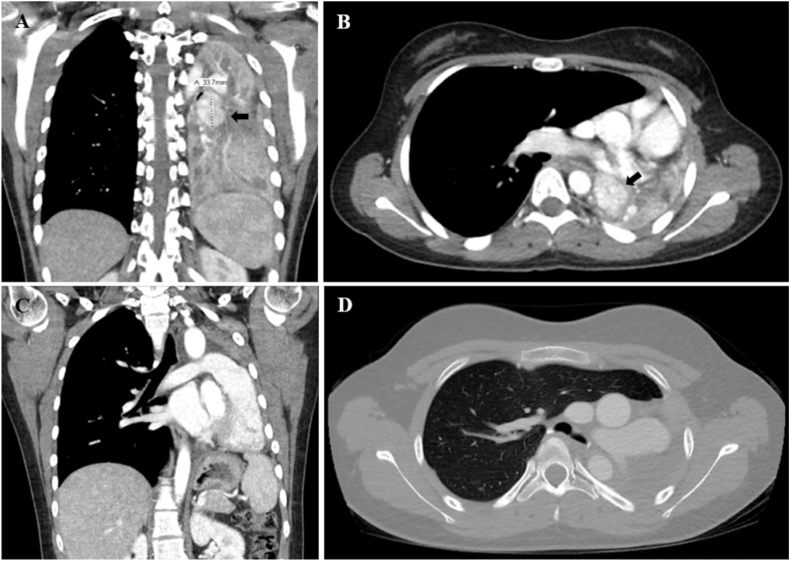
Chest CTs for case 2. A and B) Chest CT obtained prior to left pneumonectomy demonstrated a 3.4 cm × 2.4 cm x 3.3 cm enhancing round mass with complete left bronchus occlusion (black arrow), right lung hyperexpansion, and distal fluid-filled airways. C and D) Chest CT obtained 4 months after left pneumonectomy demonstrated right lung hypertrophy, left-sided shift of the heart and mediastinum, and no recurrence of the mass.
